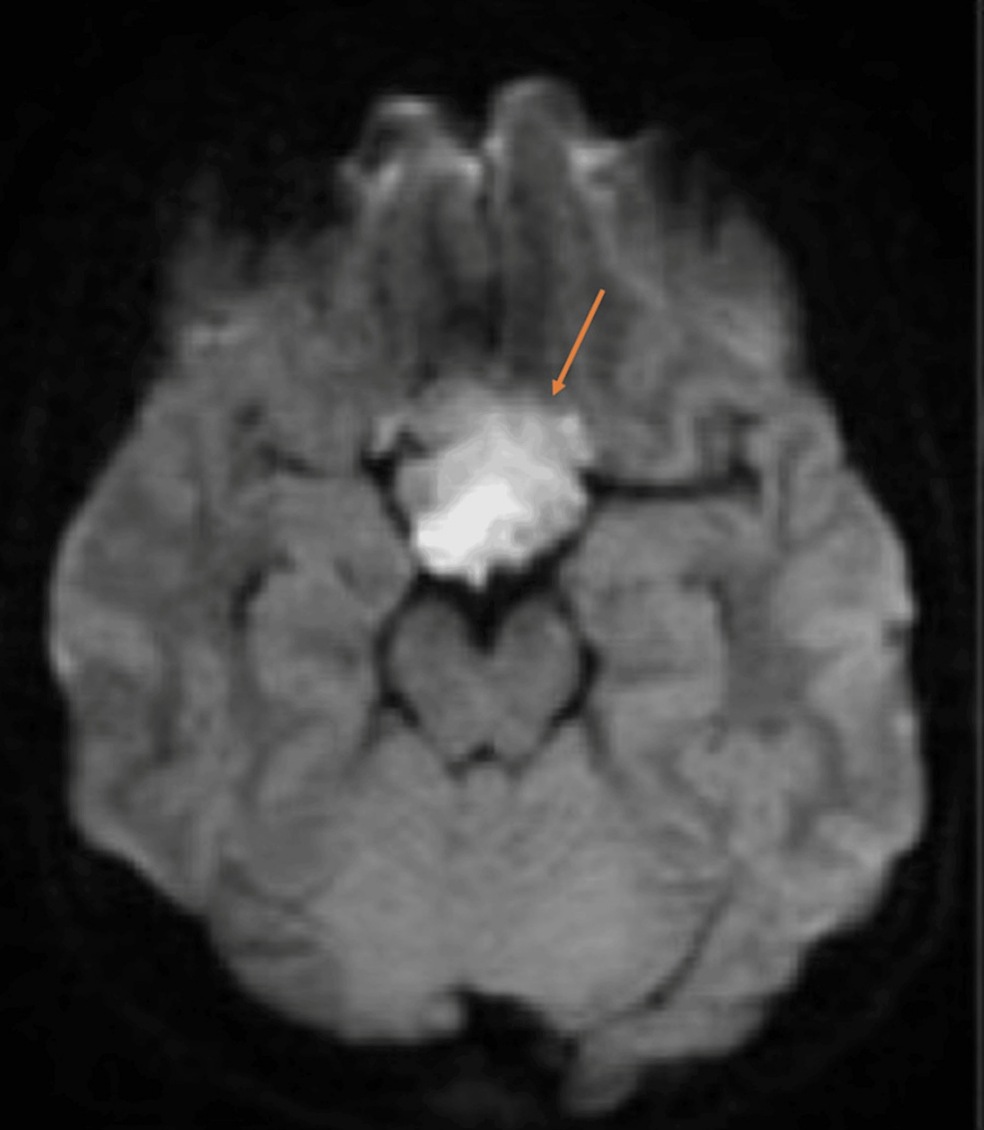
Preoperative axial diffusion-weighted image (DWI) Mild diffusion restriction with a heterogeneous nulling signal on FLAIR
